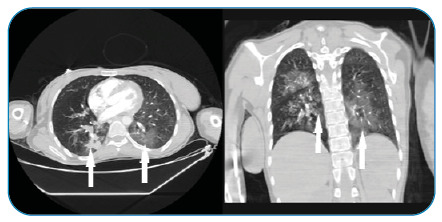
The unenhanced axial and coronal reformatted thorax CT images show peribronchovascular and subpleural ground-glass opacities and vascular dilatation (arrows) in the bilateral lung.
